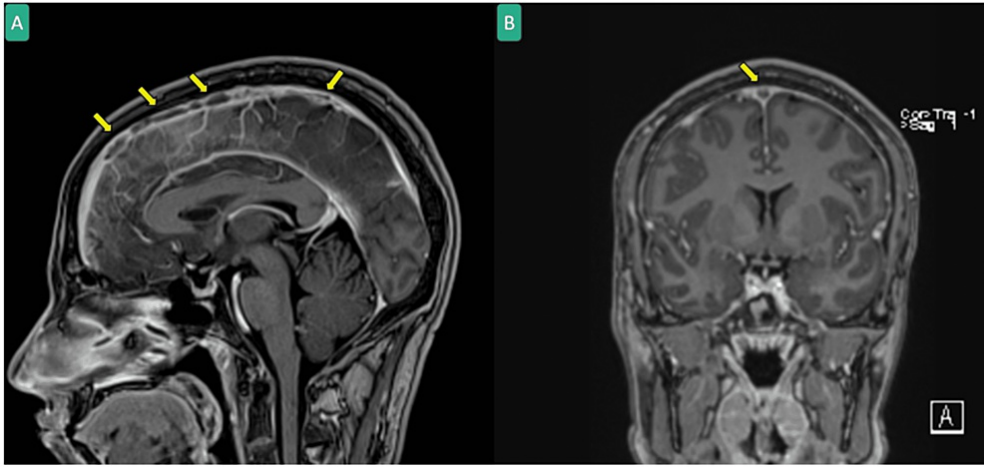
Head MRI with contrast in MPRAGE sequence sagittal (A) and coronal (B) showing extensive superior sagittal sinus thrombosis (yellow arrows). MRI: Magnetic resonance imaging
